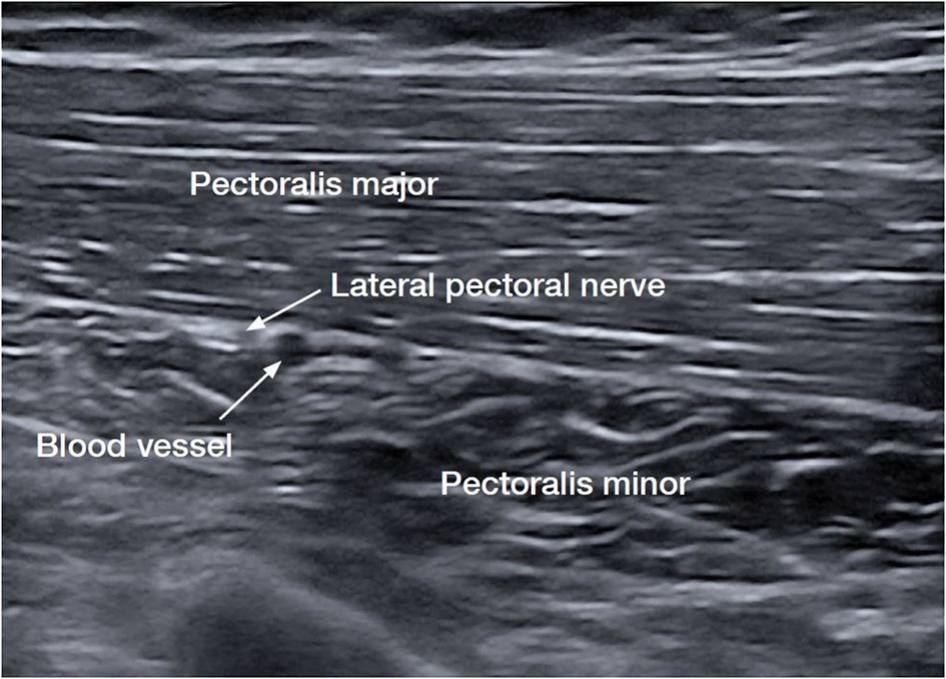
Ultrasound image of the lateral pectoral nerve (LPN), the dominant nerve of the pectoralis major muscle (PMM) (15, 16).
